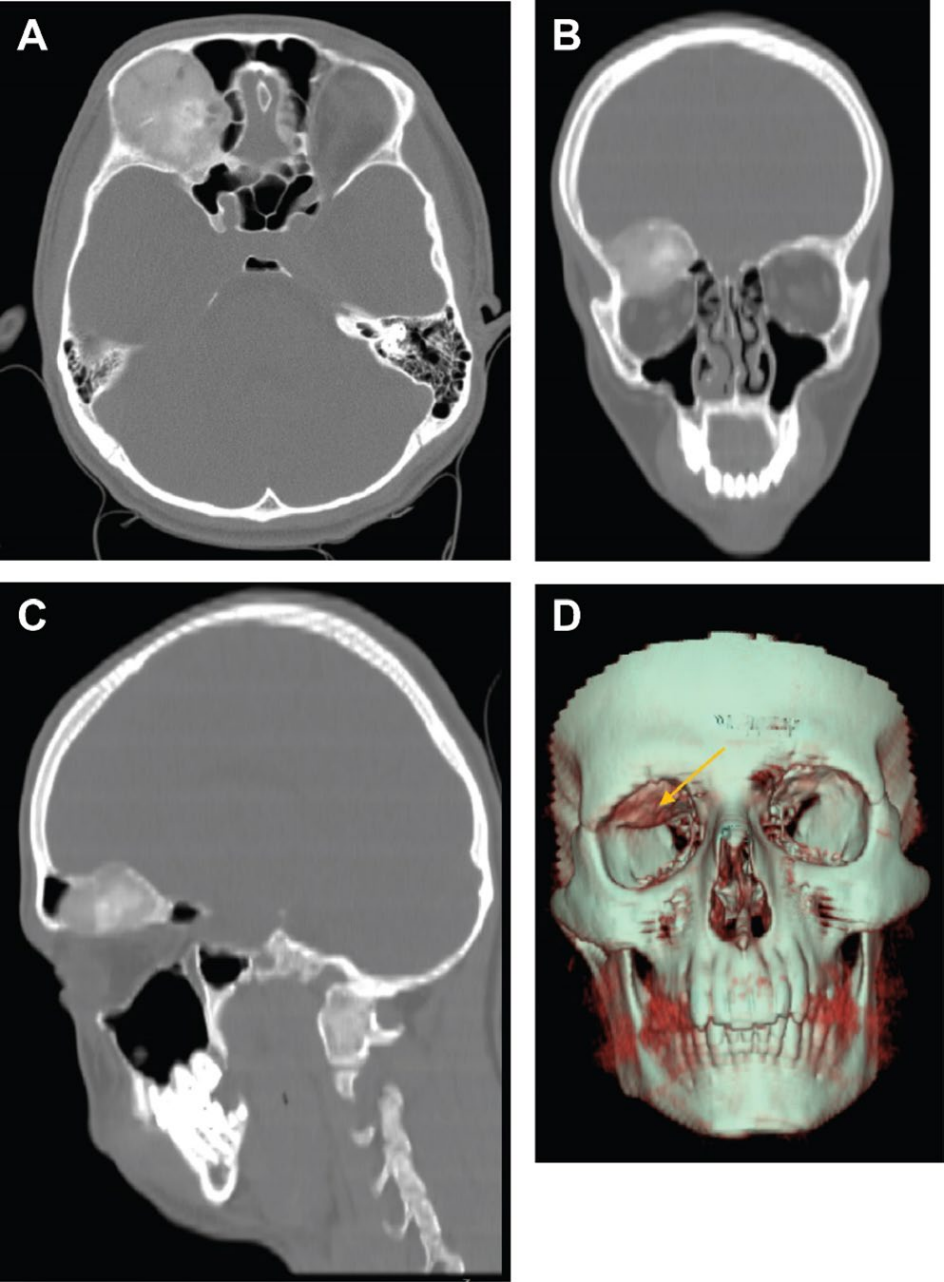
CT scans of the bony lesion. (A) Axial section displaying ground glass matrix changes of the osteoblastoma. (B) Coronal view showing the expansile bony lesion centered at the anterior cranial base and extending to involve the orbital roof. (C) Sagittal reconstruction depicting the extra‐axial lesion. (D) 3D reconstructed CT image showing expansion of the bone resulting in a decrease in the volume of the right orbit.
